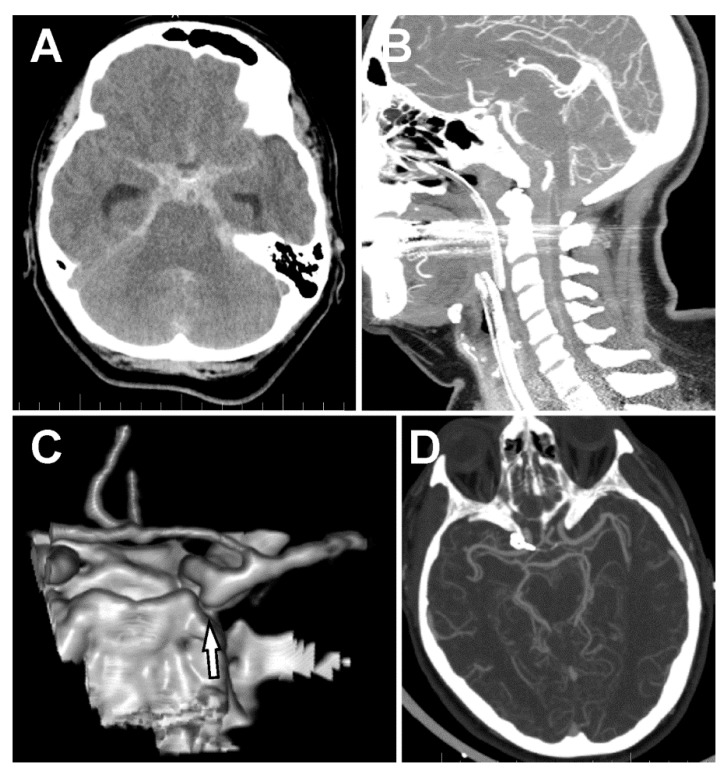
Subarachnoid hemorrhage and the posterior communicating artery (PCom) aneurysm. (A) Massive spontaneous subarachnoid hemorrhage (SAH) in the basal cisterns and both Sylvian cisterns. (B) SAH in front of the brain stem and the ventral subarachnoid space all the way down to C6. (C) 3D-CT angiography (CTA) showing an aneurysm of the right PCom artery directing downward to the posterior fossa. (D) CTA after clipping of the PCom artery.
